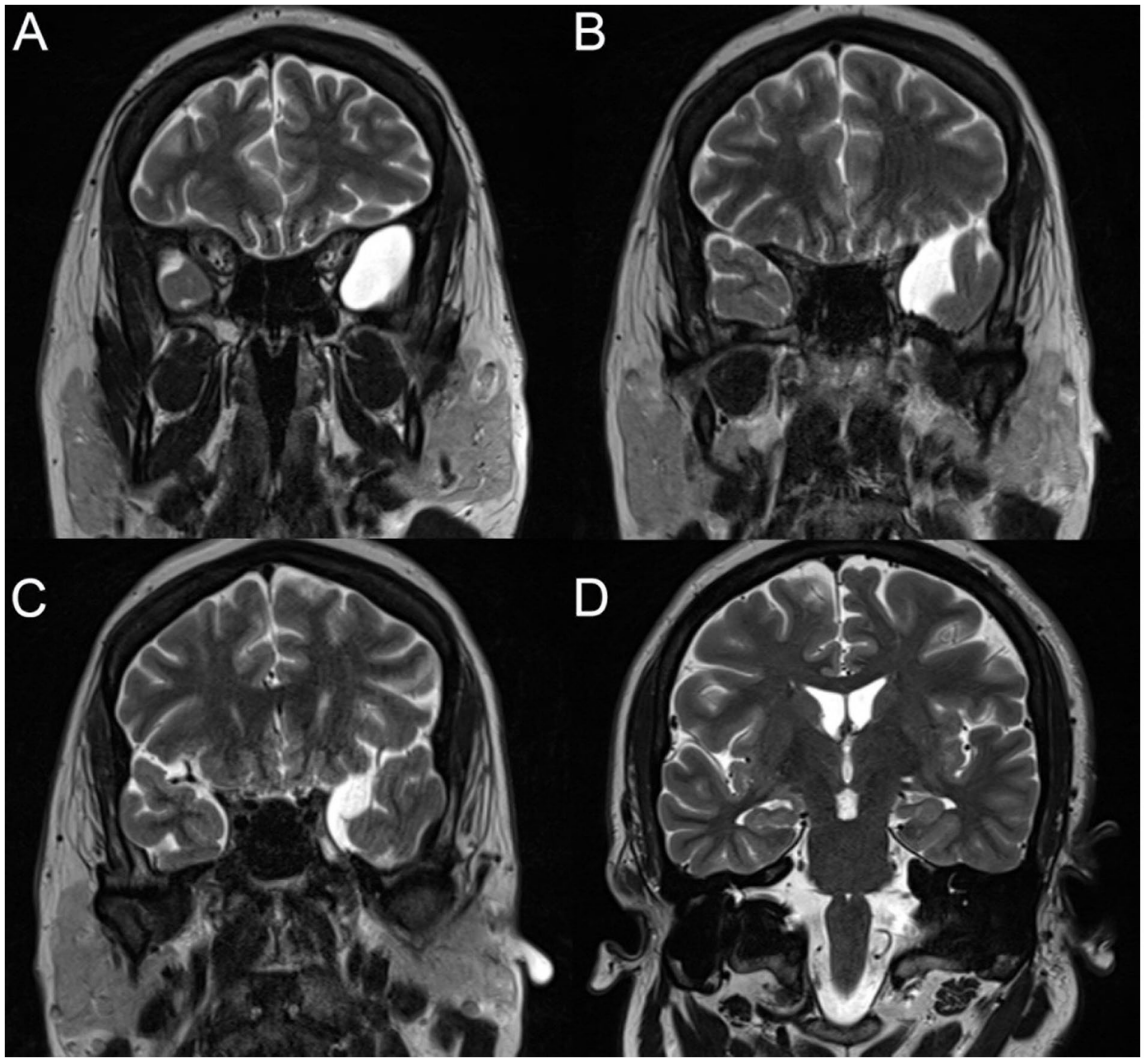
Successive T2-weighted coronal MRI of the brain showing the left temporal arachnoid cyst (A–C). The hippocampi appeared within normal limits on a more posterior cut (D).
